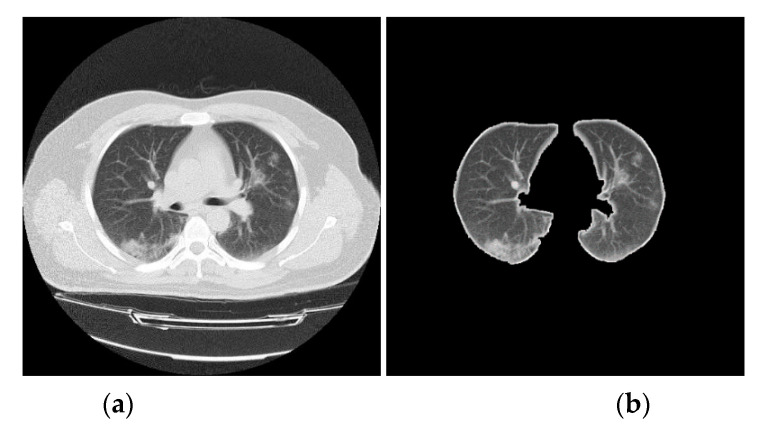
An example of lung boundary identification: (a) original CT slice, and (b) segmented CT slice.
